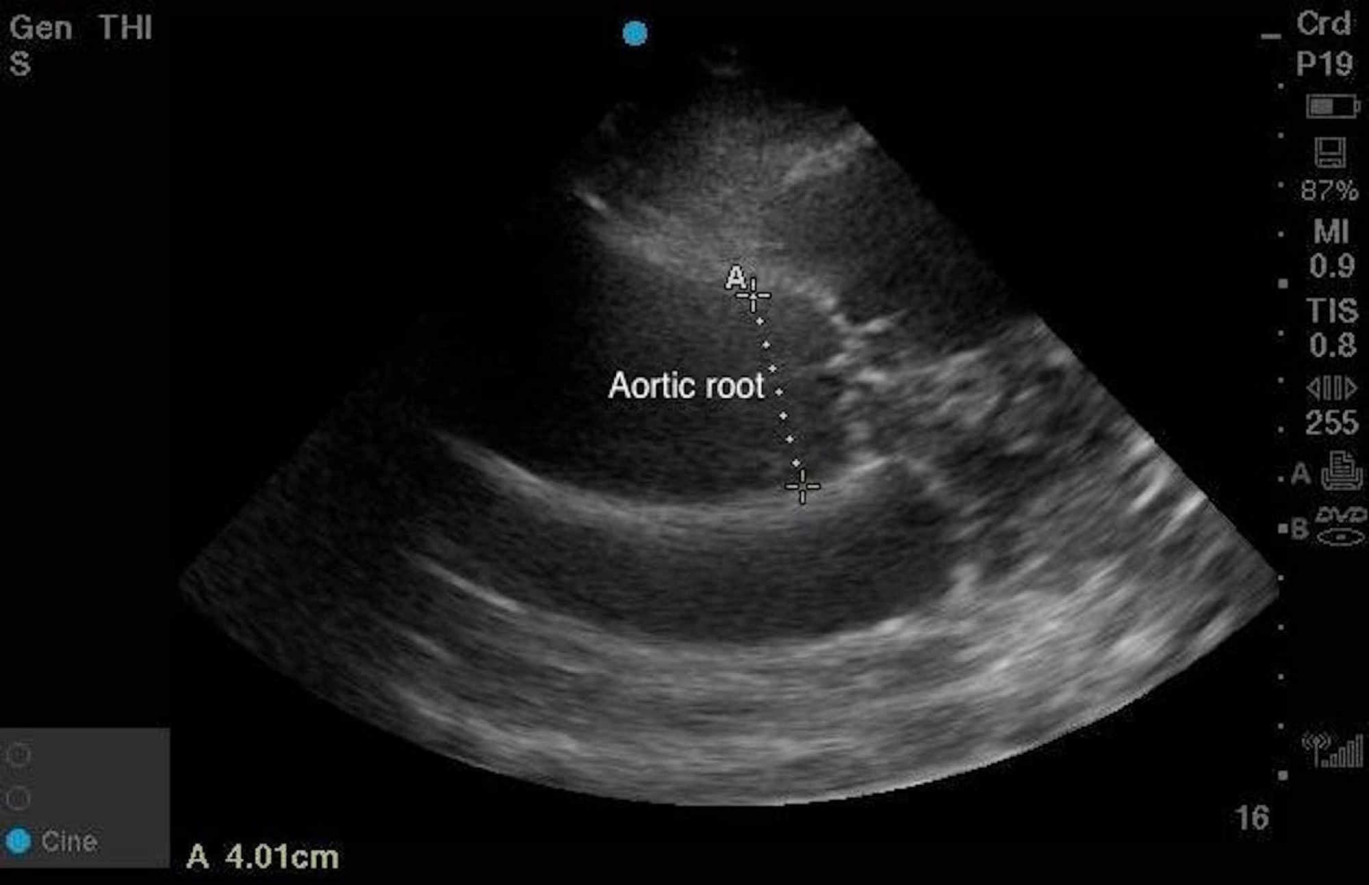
Ultrasound image of dilated aortic root
